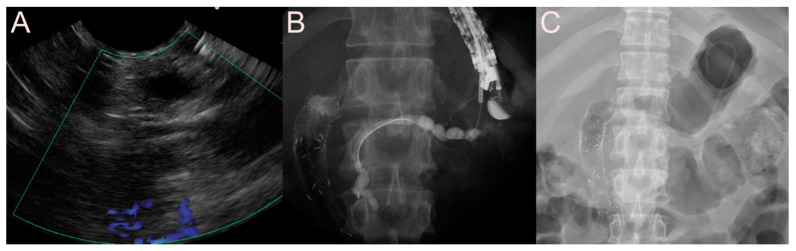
Endoscopic ultrasound-guided pancreatic duct (PD) drainage using the 7 Fr double-pigtail plastic stent (DPPS) for obstructive pancreatitis. (A) Endosonographic image showing a 19-gauge needle puncture into the dilated main PD. (B) Fluoroscopic image showing a guidewire delivered to the head portion of the main PD through a 19-gauge needle punctured into the upper site of the stomach. (C) Fluoroscopic image showing the 7 Fr DPPS placed between the main PD and the stomach.
